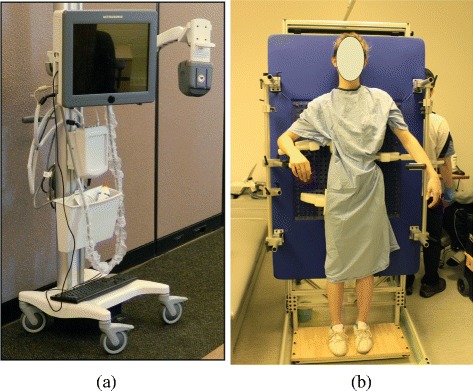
The apparatus for patient data acquisition. a) The 3D ultrasound equipment (Left) and b) the set up to scan a brace subject.
